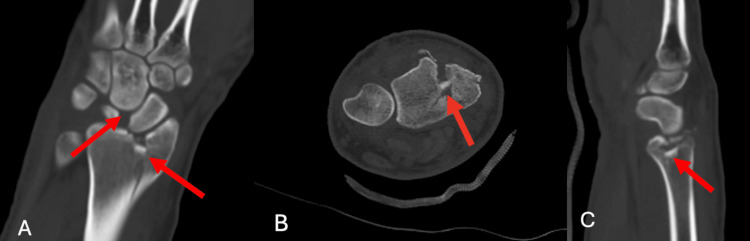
Preoperative Computerized Tomography of the Right Wrist Preoperative CT coronal (A), axial (B), and sagittal (C) imaging of the right wrist after a closed reduction demonstrates the comminuted fracture of the distal radius with a residual scapho-lunate diastasis. There is collinearity between the distal radius and carpal bones (red arrows).
